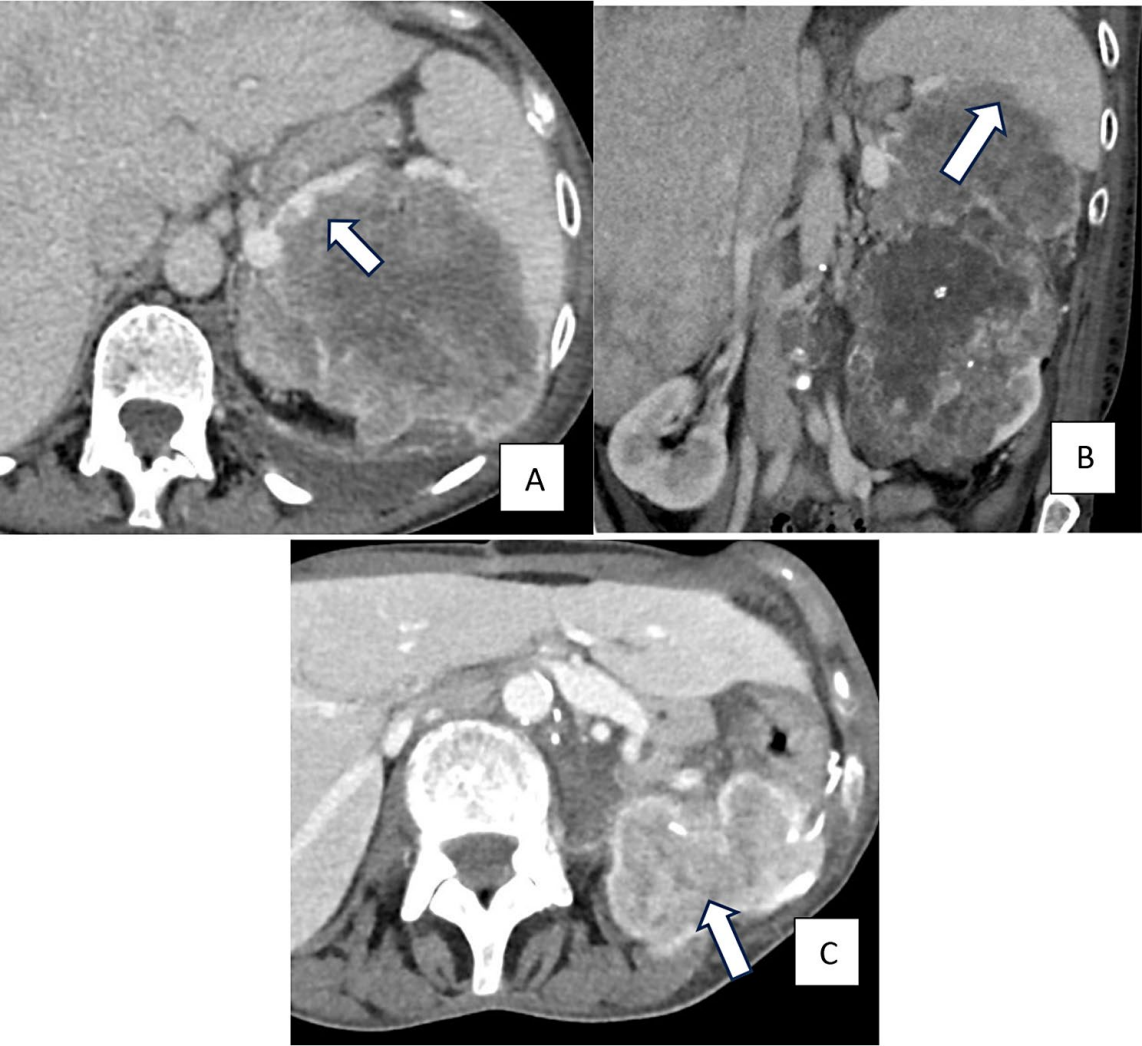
Contrast-enhanced axial (A) and coronal CT images (B) of the abdomen at the level of the left kidney show a large heterogeneous mass with extensive sarcomatoid differentiation invading the left kidney, left adrenal gland, distal pancreas and spleen. (C) Post-operative contrast-enhanced axial CT of the abdomen at the level of the left kidney shows local recurrence in the surgical bed
